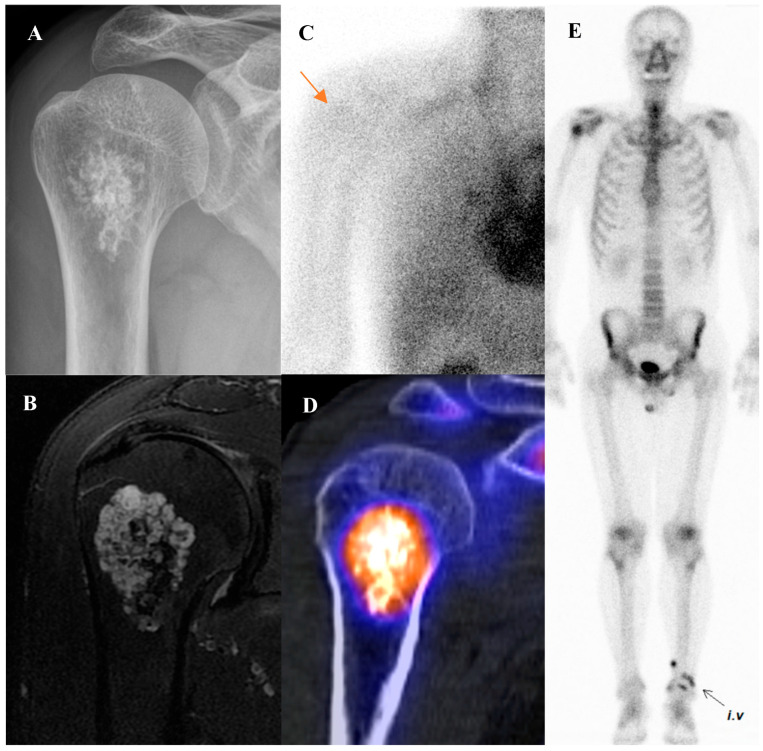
A 58-year-old male with enchondroma of the right humerus. (A) Plain radiograph shows popcorn-like calcifications at the humeral neck. (B) T2-weighted fat-suppressed magnetic resonance imaging demonstrates a lobulated intramedullary tumor in the proximal metaphysis, showing predominantly high signal intensity with focal low to intermediate signal areas. (C,E) Bone scintigraphy reveals mildly increased perfusion on the blood pool phase (arrow) and moderate uptake on the delayed phase at the lesion site. (D) Bone single-photon emission tomography/computed tomography demonstrates increased uptake at the proximal humeral metaphysis, corresponding to the calcified lesion.
